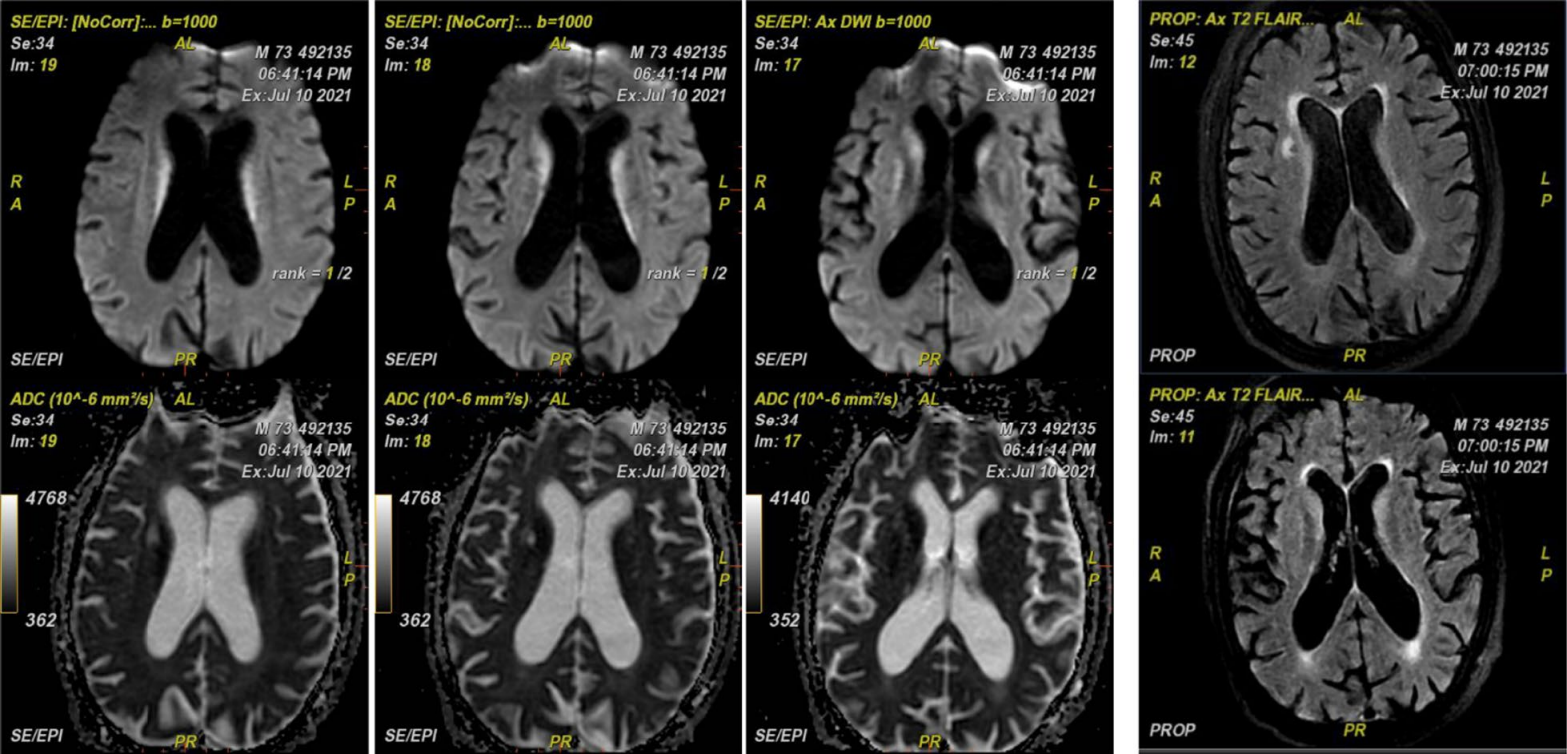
Conventional MRI data with diffusion images showing abnormal relative diffusion restriction with faint abnormal high signal on FLAIR, at the deep gray matter nuclei particularly caudate nuclei and anterior aspects of the putamen and thalamic nuclei
